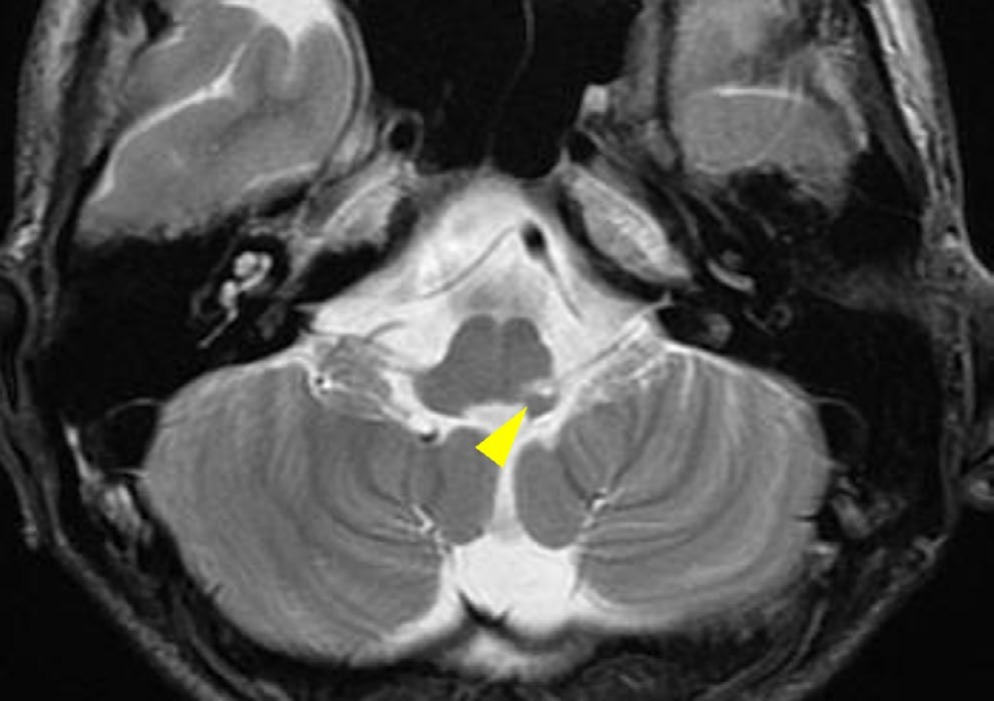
Magnetic resonance imaging 14 mo after onset. T2‐weighted image showing high intensity and confined to a small region of the left dorsolateral medulla (yellow arrow)
